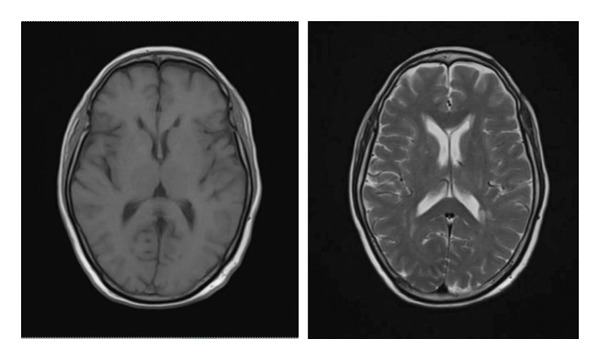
The cranial MRI on the 10th day after initial symptoms.
